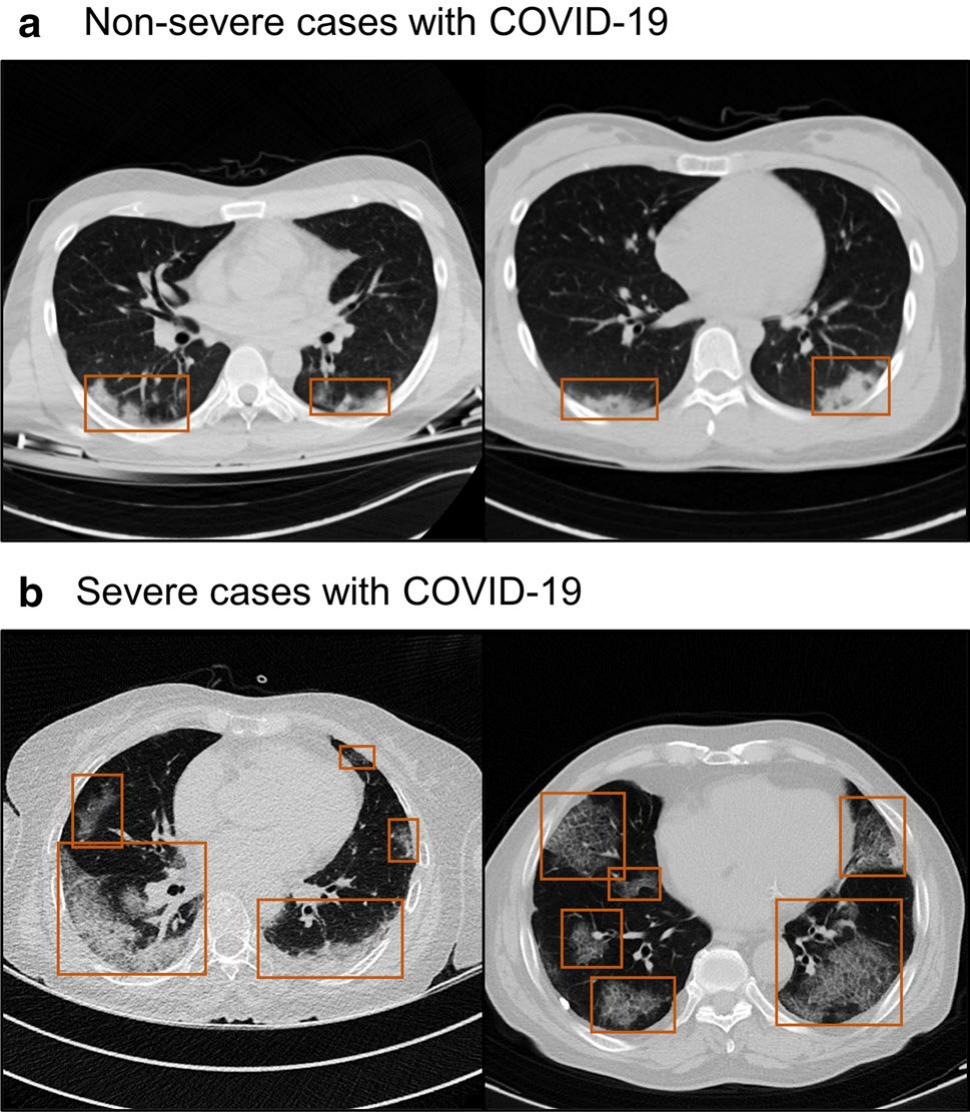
Example CT images with COVID-19. (a) Non-severe cases. (b) Severe cases. COVID-19, coronavirus disease 2019.
